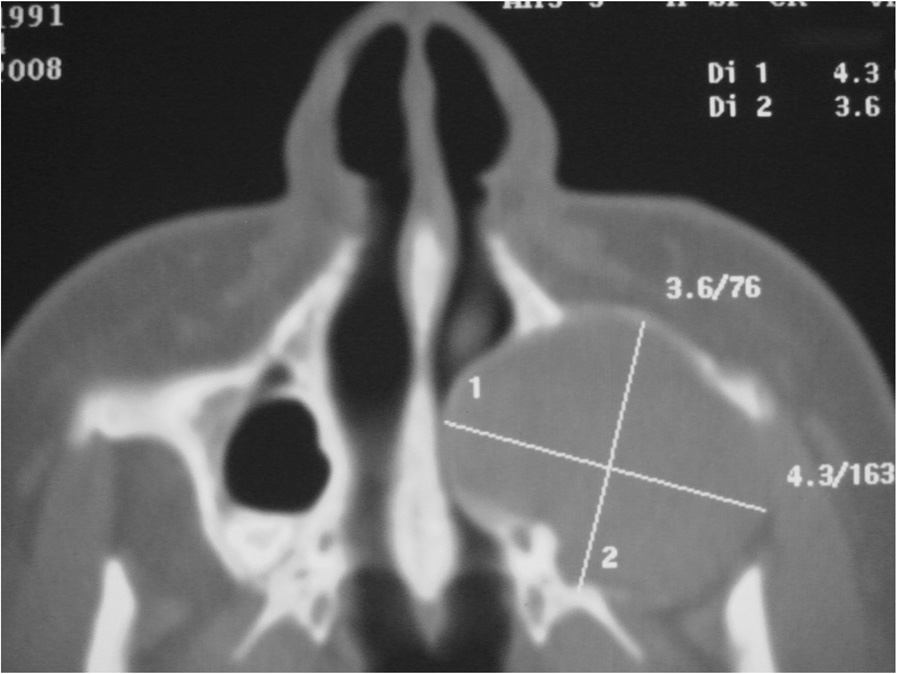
Computed tomography scan: axial sections.
